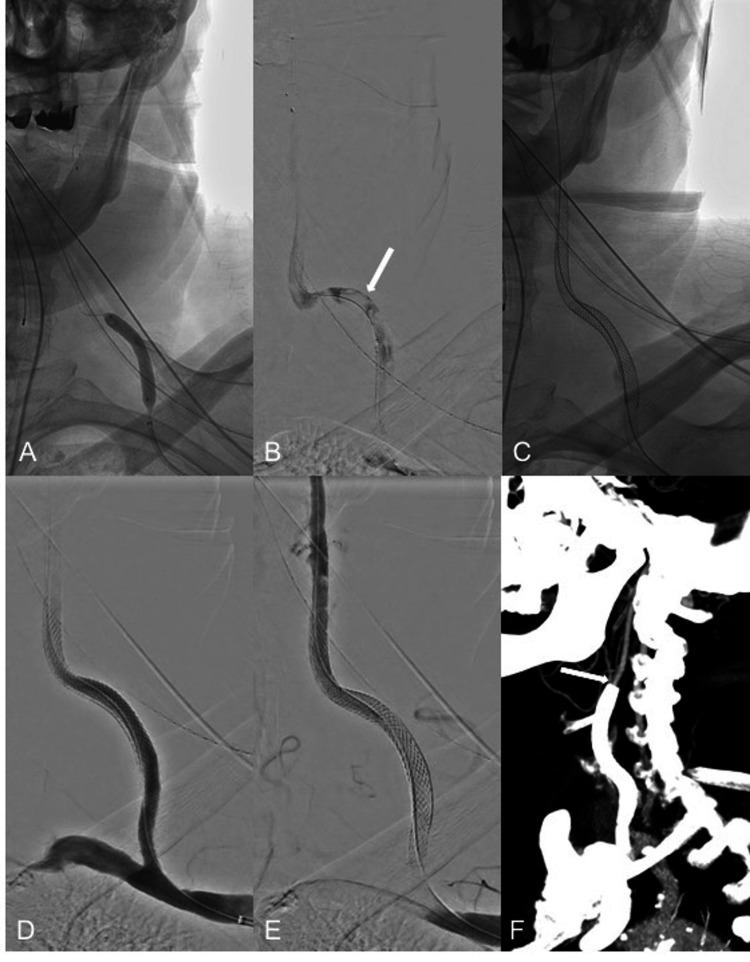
Bypass Graft Recanalization Multiple overlapping angioplasties are performed along the entire length of the bypass graft (A). Persistence of high clot burden throughout the entire graft is seen (B, arrow). Placement of three overlapping self-expanding carotid stents including a balloon-mounted stent at the site of the proximal anastomosis of the graft (C). Final angiogram images show recanalization of the bypass graft (D and E). Ten-month follow-up CT angiogram shows a patent bypass graft (F, arrow).
